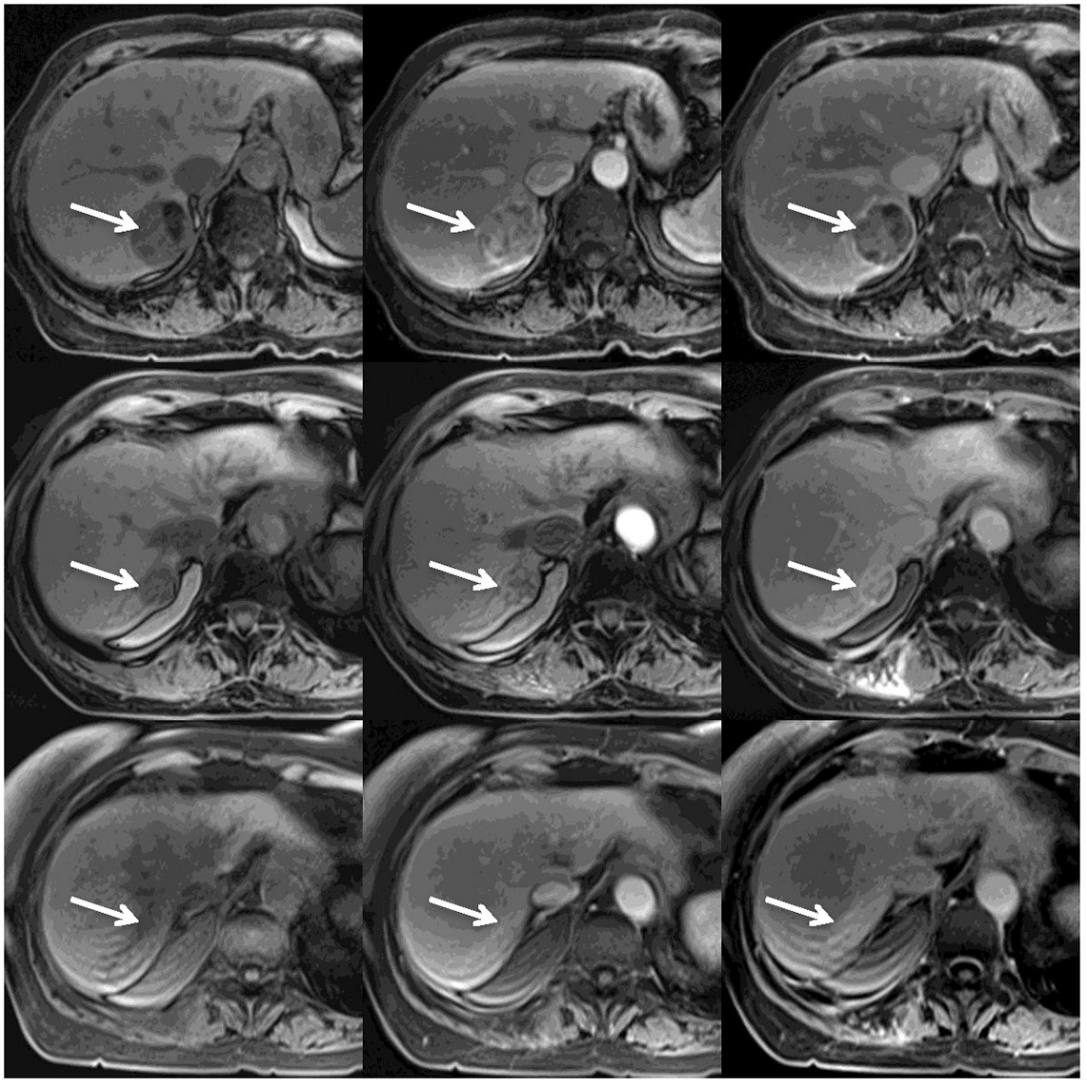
MRI Treatment Response Figure 3: Serial MRI images obtained before (upper row), 3 months after (middle row) and 39 months after (lower row) the SBRT treatment.
